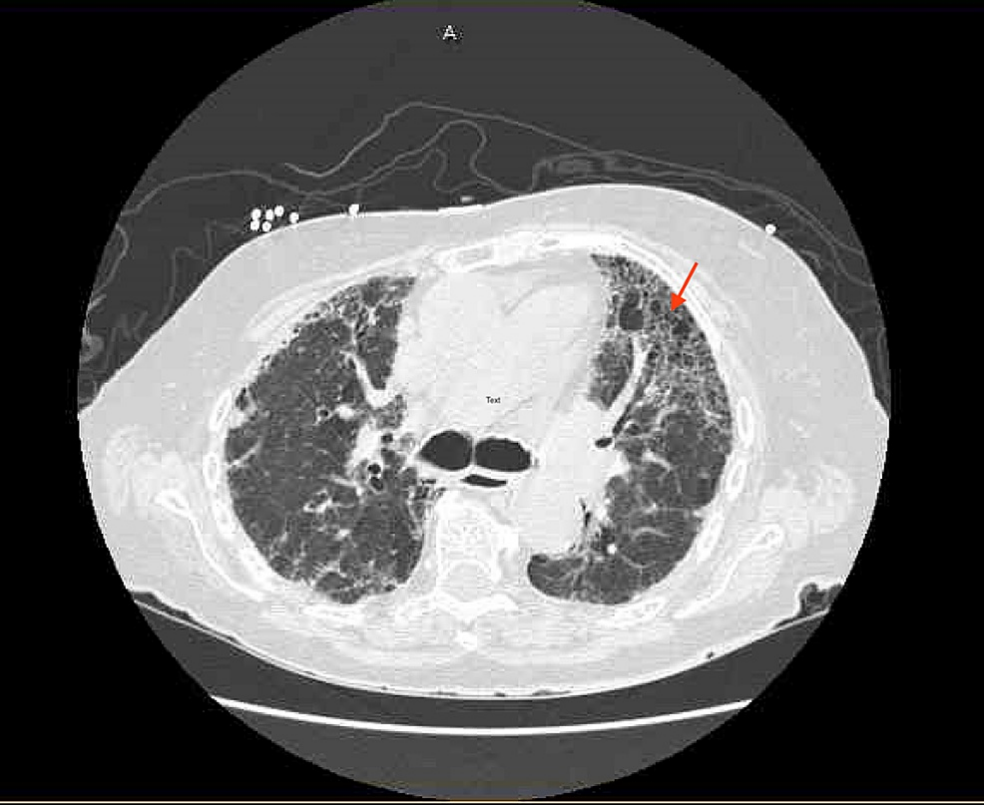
Chest CT without contrast showing honeycombing-lesion of pulmonary fibrosis (red arrow).
